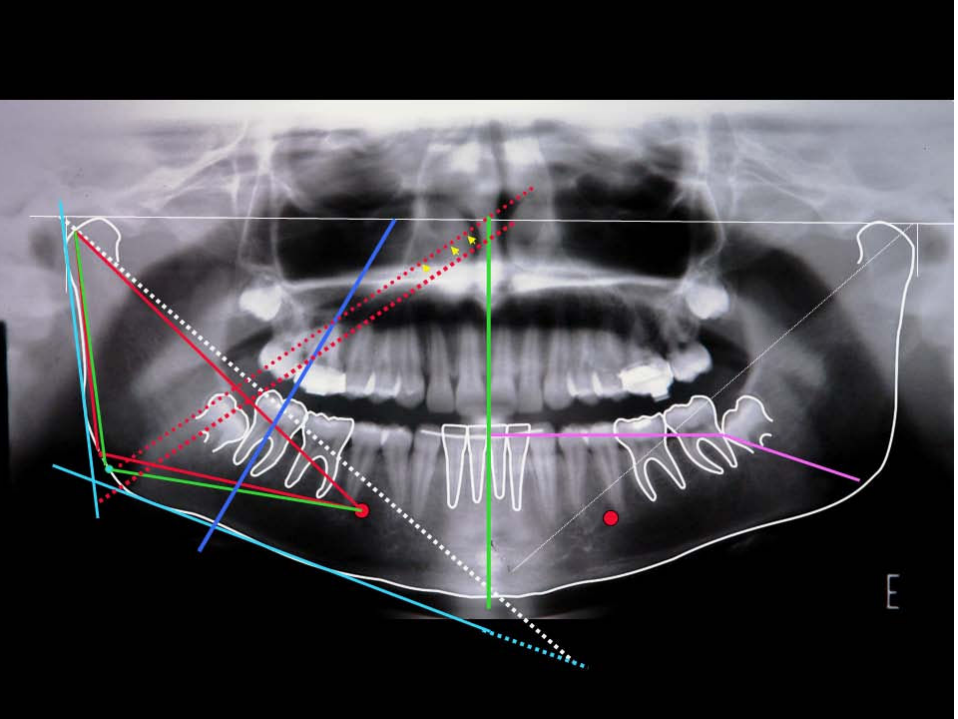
Image of panorametry traced over a panoramic radiography with information for bilateral bone-dental angular measurements of the mandible. For clarity, the different possibilities described in the text are not presented (they may be seen in the preceding figures). Besides different linear or angular measurements, the triangular areas may also be measured and compared in terms of surface. See adequacy of 48 AX (arrows) for determination of its angle with HRP. We also point out the possibility of linear intercoronaly measurements, such as M 38- ML (90°) and M 38- l MPGo (pink).
